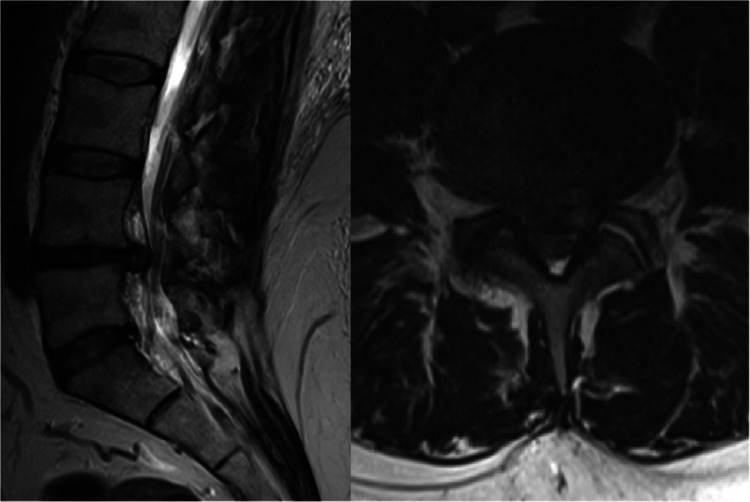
Preoperative sagittal and axial T2 MRI demonstrating a diffuse central disc bulge causing severe spinal canal stenosis with bilateral L5 nerve root compression
